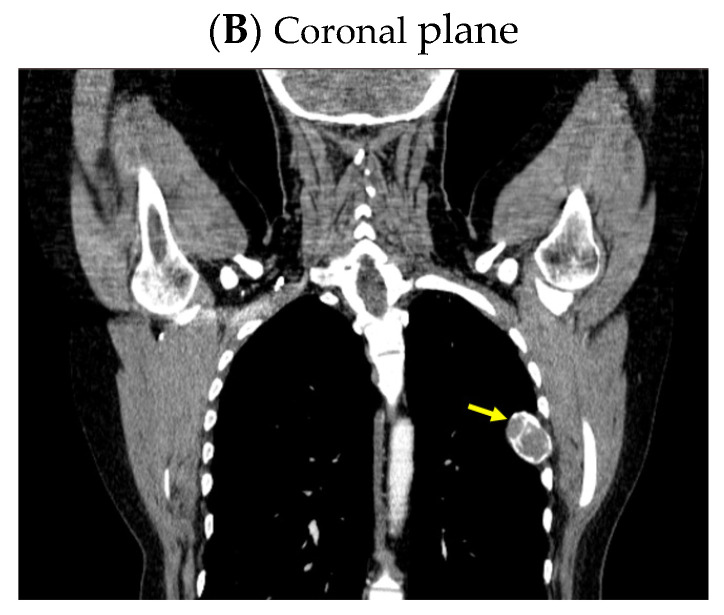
Intravenous contrast CT: a tumor mass located in the middle and posterior part of the left sixth rib, with median cortical destruction of 2.53 by 3.31 cm, suggestive of a brown tumor (yellow arrow).
